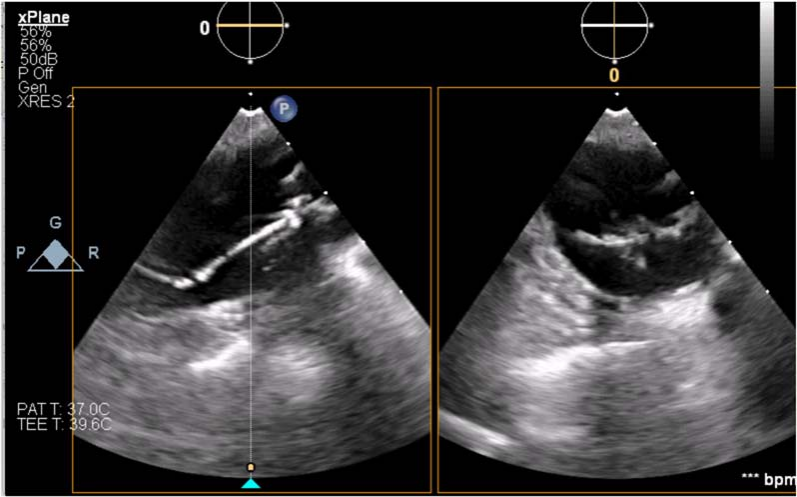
Transesophageal image of the ascending and descending aorta with mispositioned IABP present.
